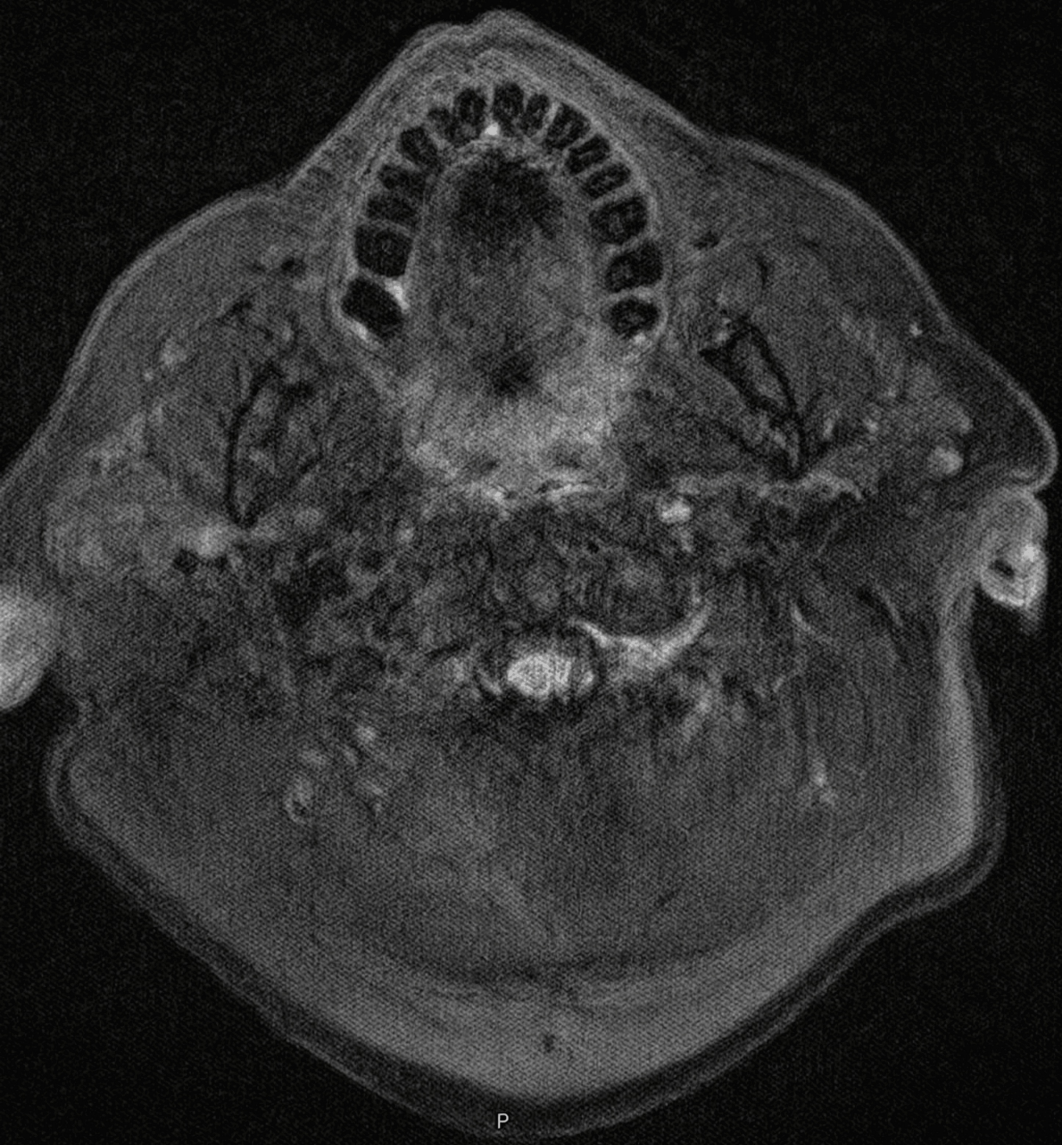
MRI of the brain showing no brain metastasis (T2-weighted FLAIR on an axial view) MRI: magnetic resonance imaging; FLAIR: fluid-attenuated inversion recovery
